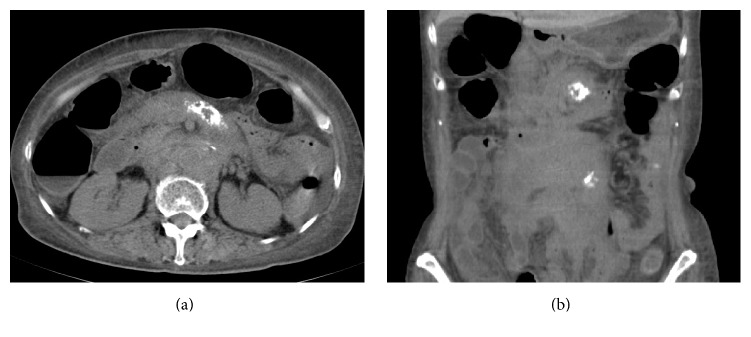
Abdominal computed tomography showing irregular soft tissue tumor masses with calcification in the abdominal aortic retroperitoneum and mesentery.
